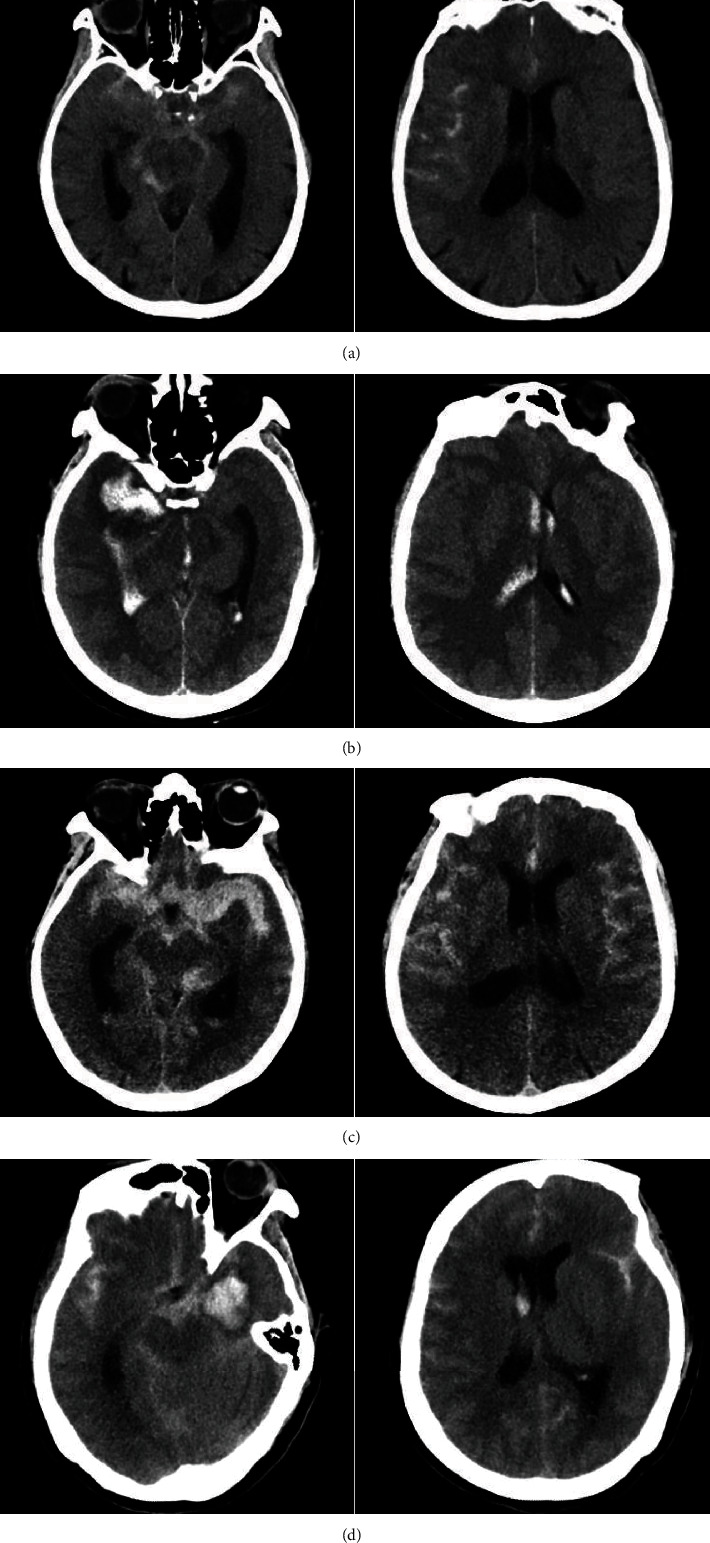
The mFS, a CT-based rating scale is as follows; (a) Grade 1 consists of thin, minimal, or diffuse SAH without IVH; (b) Grade 2 consists of minimal or thin SAH with IVH; (c) Grade 3 is defined by a thick cisternal clot without IVH; (d) Grade 4 is defined by a cisternal clot with IVH. Abbreviations: CT: computed tomography; IVH: intraventricular hemorrhage; mFS: modified Fisher scale; SAH: subarachnoid hemorrhage.
